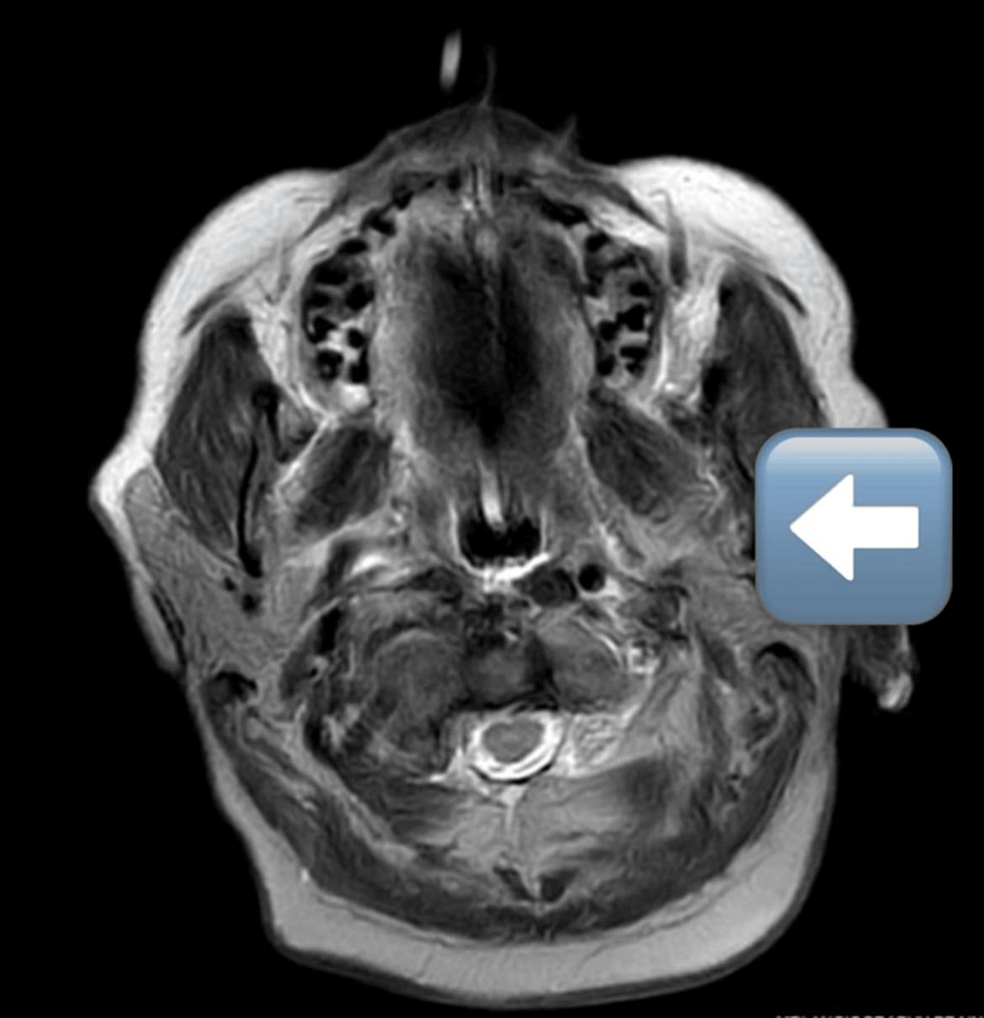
MRI brain impression reveals acute non-hemorrhagic infarcts involving bilateral cerebellum, dorsal pons, ponto-mesecephalic junction on the right side, and bilateral occipito-parietal cortex.
